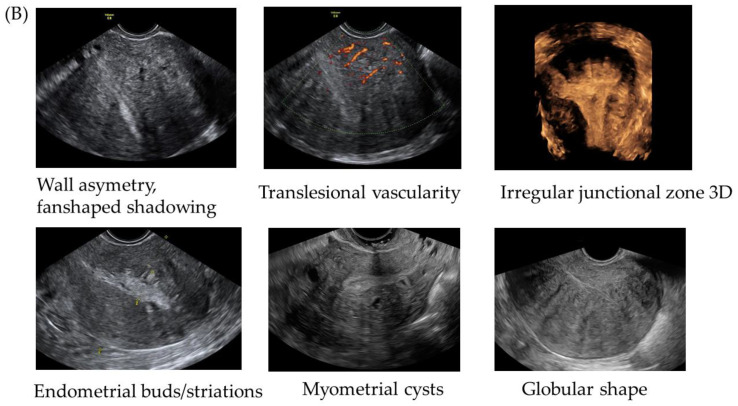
(A,B). TVS measurements and features in women with adenomyosis. (A) Measurement of (a) uterine anterio-posterior diameter, (b) anterior-, and (c) posterior uterine wall thickness in the longitudinal plane. Junctional zone (white arrow), measurement inthe 3D reconstructed coronal plane. (B) Examples of sonographic features of adenomyosis.
